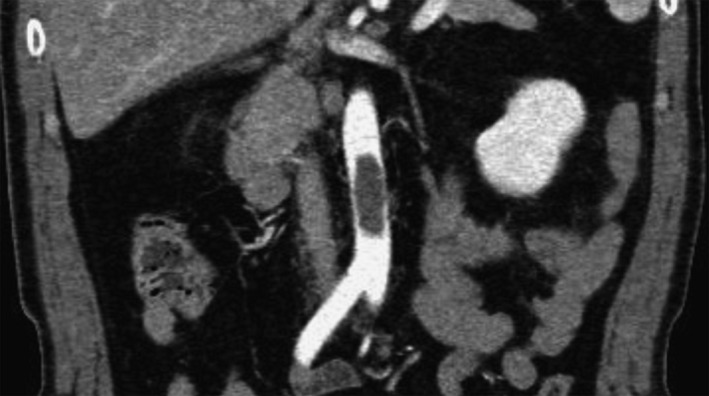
Enhanced computed tomography showing a 4 × 1.5 × 1.5 cm egg‐shaped thrombus in the abdominal aorta
